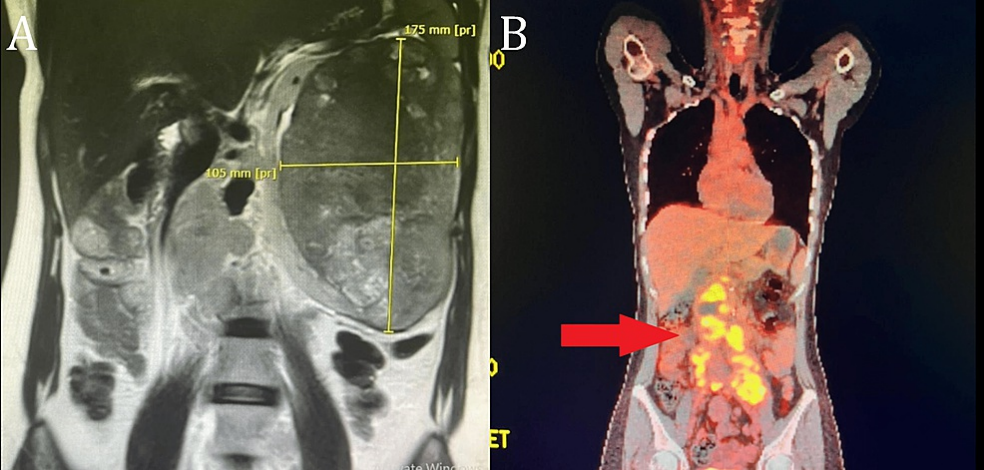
(A) Renal MRI Vefore Left Radical Nephrectomy; (B) Baseline PET/CT Scan Before ICI Treatment. (A) Left heterogeneous enhancing renal mass measuring (175x105 mm). (B) Numerous retroperitoneal lymph nodes involving left para-aortic, aortocaval, and retrocaval lymph nodes. ICI: immune checkpoint inhibitors
